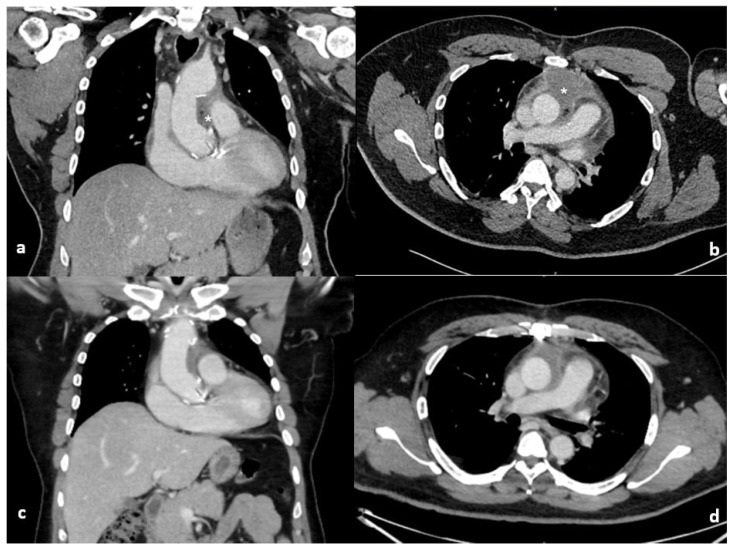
Coronal and axial computed tomography of the chest on the 10th (a,b) and 27th (c,d) post operative days. The initial large loculated collection with blebs of air and enhancing walls (*) surrounding the ascending aorta has improved with conservative management on repeat imaging.
